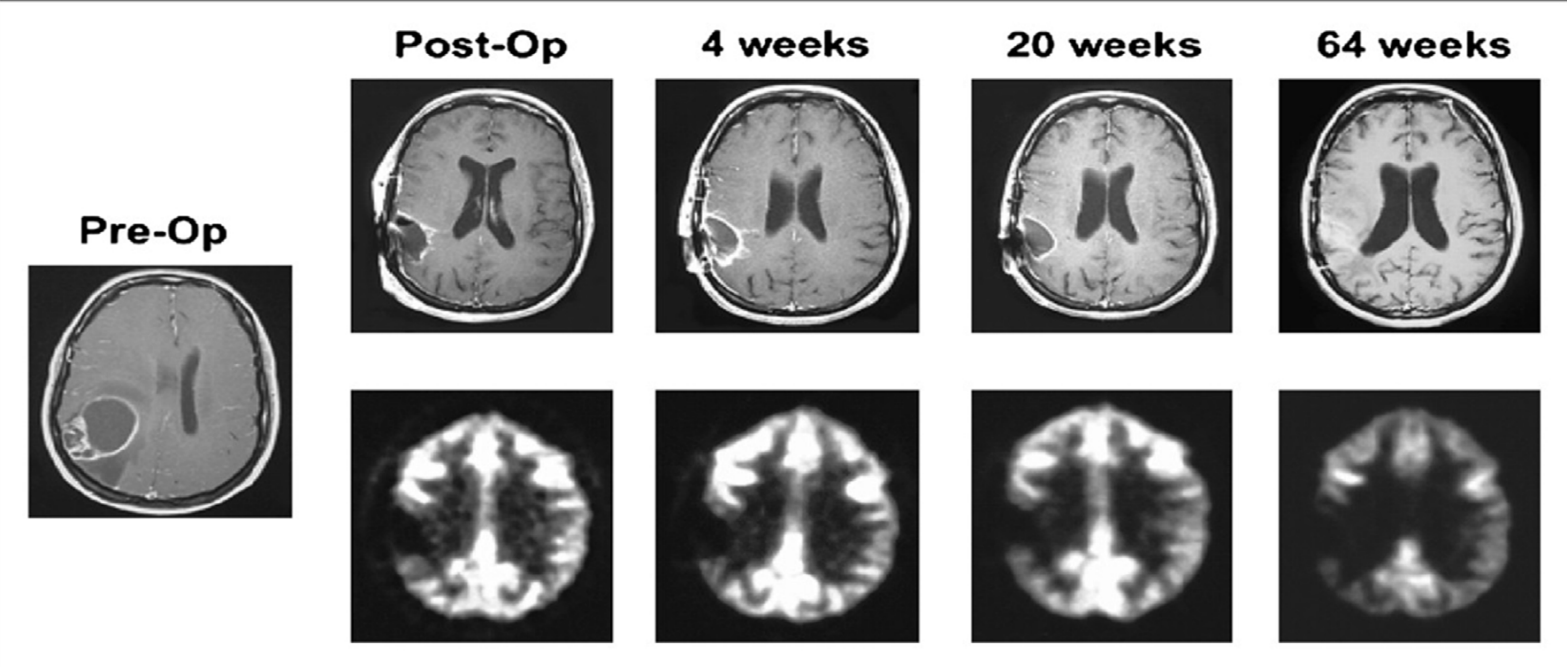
Serial MRI (top and middle) and ^18^F-FDG PET scan results of representative patient after ^131^I-ch81C6 therapy. Corresponding ^18^F-FDG PET scan images (bottom) demonstrate a lack of increased metabolic activity in region of surgically created resection cavity. *This research was originally published in JNM* (Reardon et al., 2006b).
